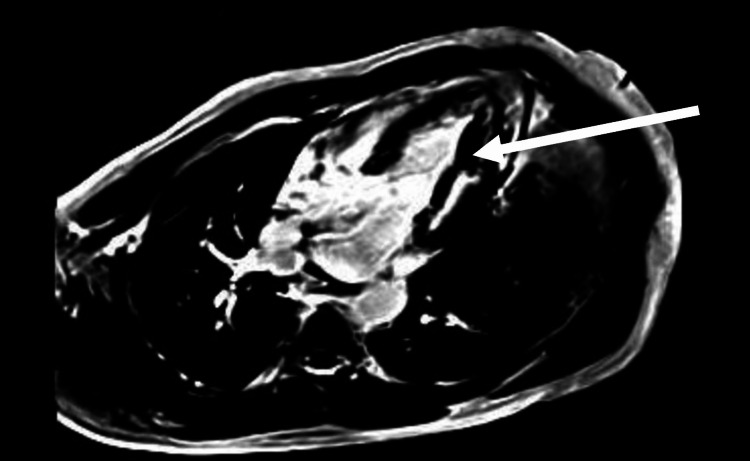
Phase-sensitive inversion recovery (PSIR) MRI image showing a layer of thrombus overlaying the lateral left ventricle.
